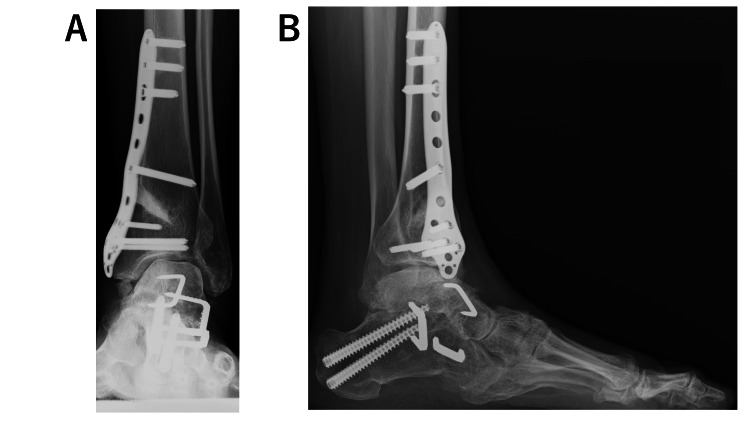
Plain radiographs of the left foot obtained 4 years after the revision surgery. (A, B) Some residual β-tricalcium phosphate can be seen remained in the in the frontal view, but bone fusion was complete.
